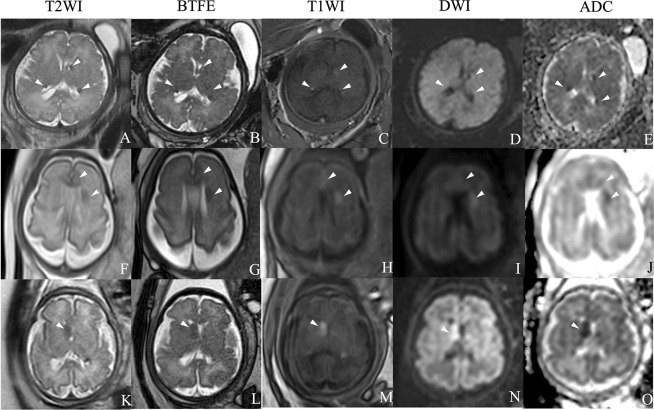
TSC-related MRI characteristics on fetal brain MRI. Cortical dysplasia appear as hyperintense signals on T1WI against the dark background of the white matter, a phenomenon often referred to as the "bright spot" sign. The white arrow represents the lesion. (A–E) Subependymal nodules; (F–J) cortical dysplasia (includes cortical tubers and white matter migrational abnormalities); (K–O) subependymal giant cell astrocytoma.
